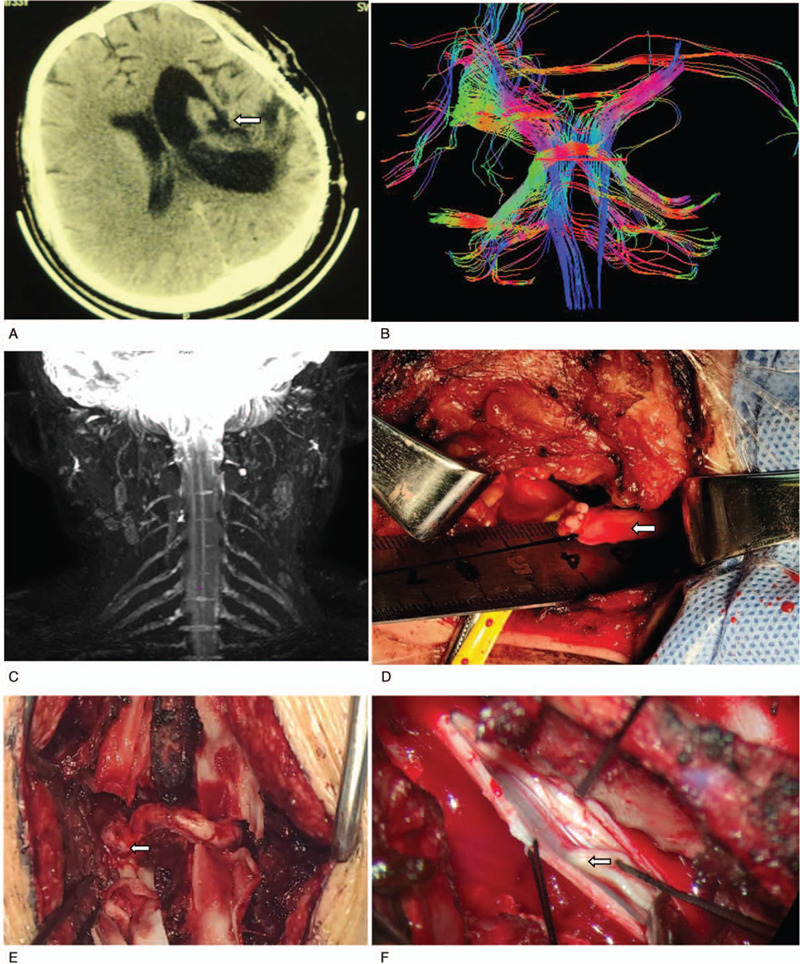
A: Preoperative head computed tomography (CT) scan revealing a softening lesion of the left basal ganglia. B: Diffusion tensor imaging (DTI) of the head showing a significant decrease in fibers in the left basal ganglia. C: Magnetic resonance imaging (MRI) scan of the brachial plexus, revealing normal bilateral brachial plexuses. D: Cutting and measuring the CC7 nerve. E: Tension-free anastomosis between the left C7 nerve and the right one. F: Exploring the posterior root of the C6 nerve.
